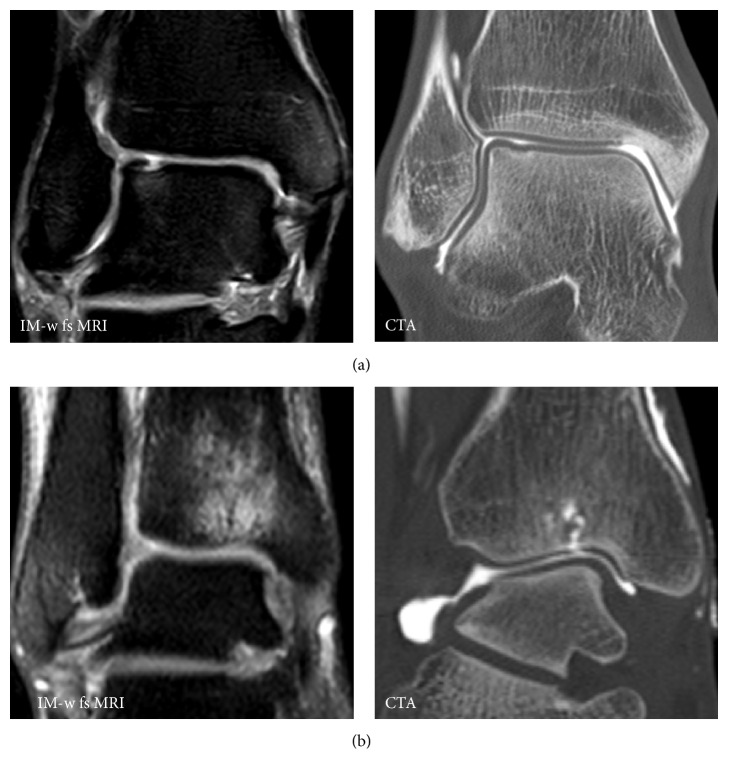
Divergent results for presence of full thickness cartilage defects on MRI and CTA. In case (a) a full thickness cartilage defect at the talus was suggested on MRI; CTA revealed depression of the cartilage surface but no full thickness defect. In case (b) no cartilage defect was suggested on MRI but due to massive bone marrow edema at the tibia CTA was performed and revealed a fissural full thickness defect at the tibia.
